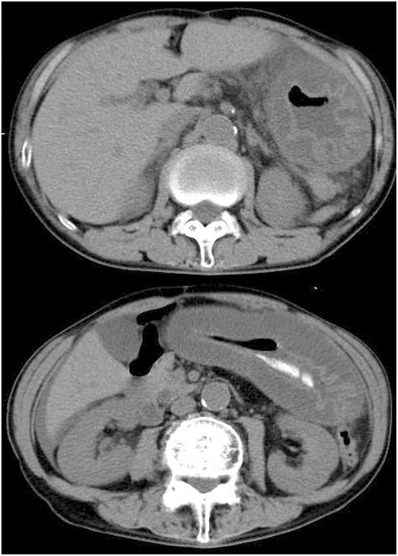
Images of abdominal computed tomography and upper gastrointestinal endoscopy
